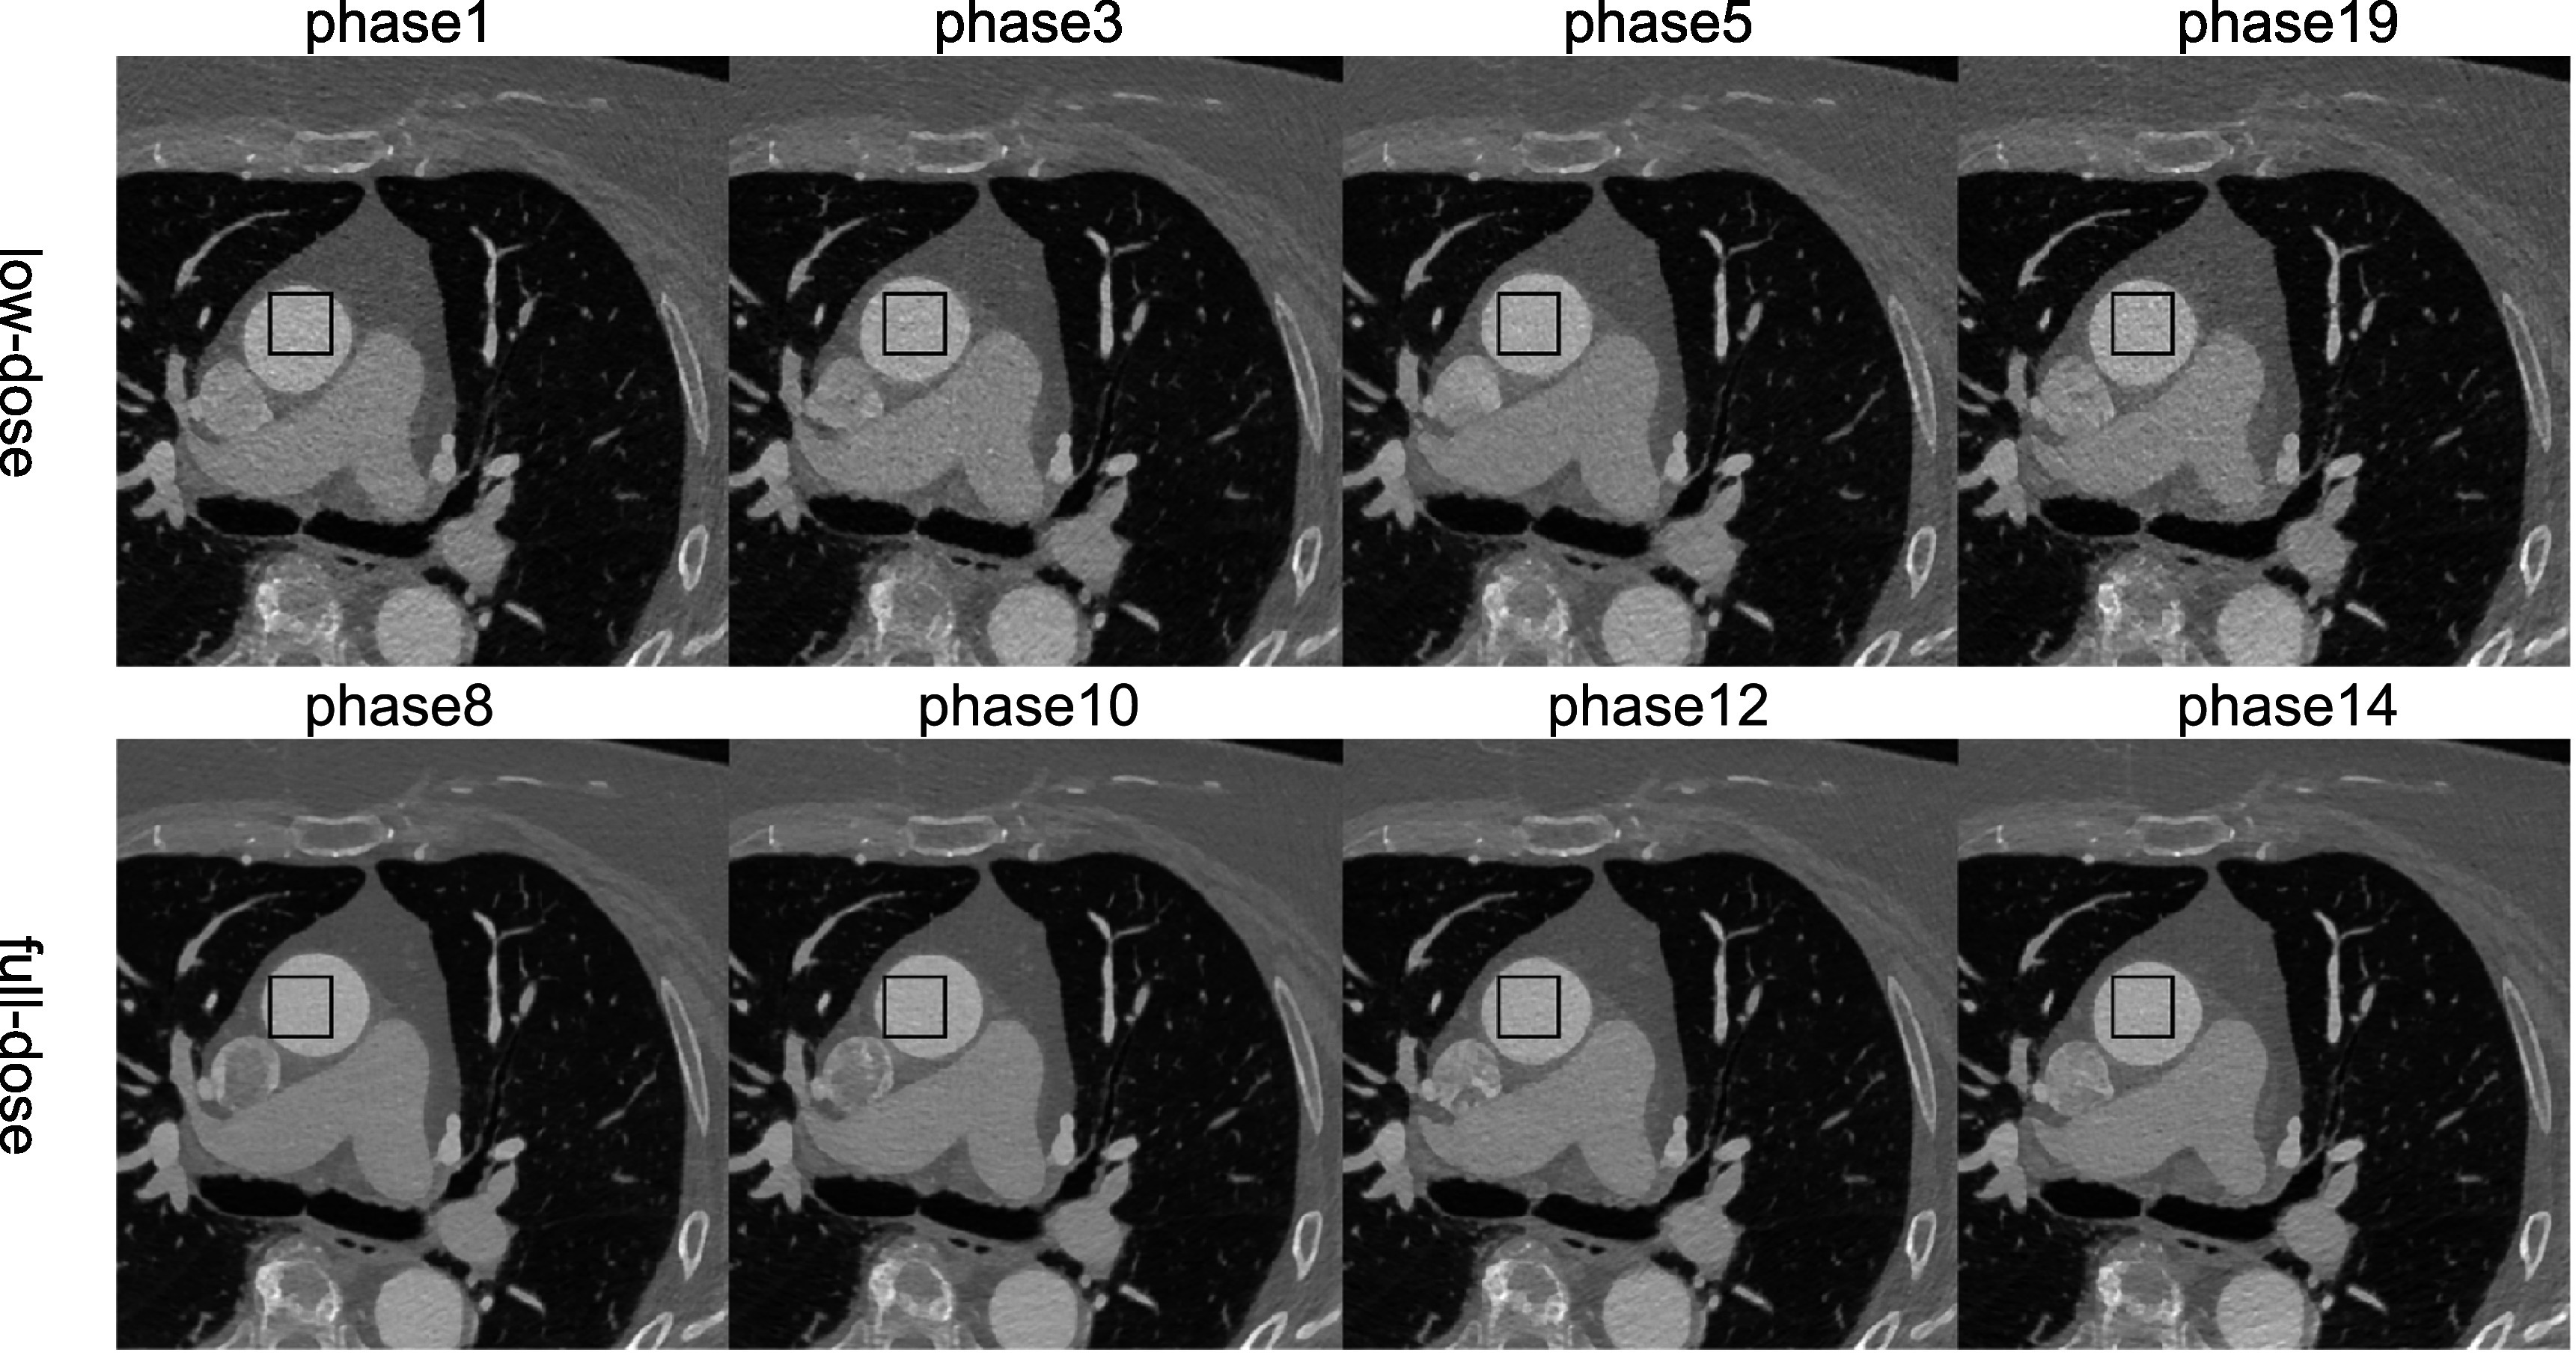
Twenty phases of the test patient CTA images (Display Window [−1000 950]HU).
